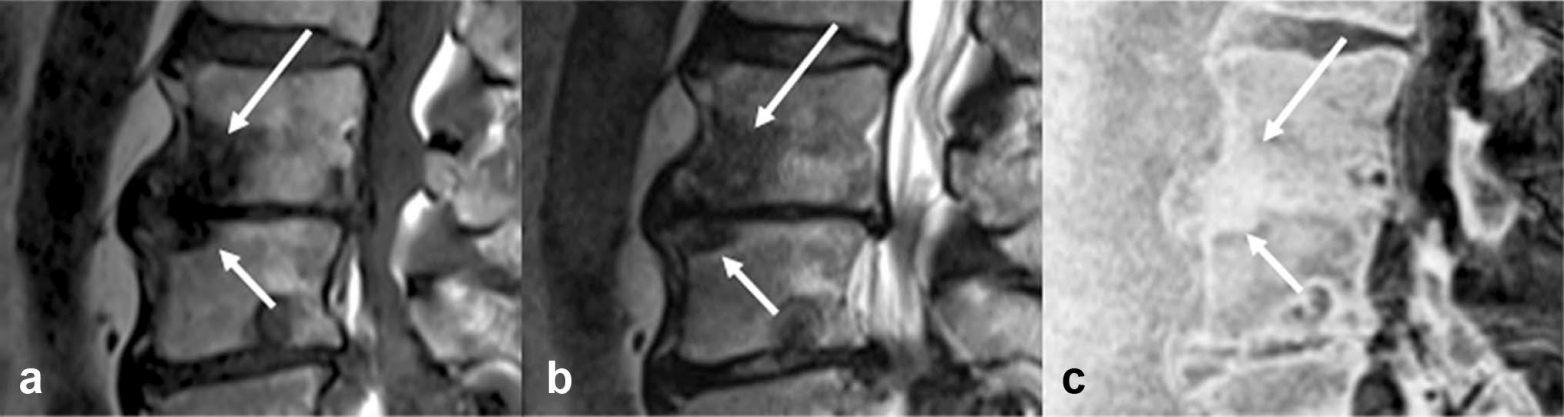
Modic type 3 change. Modic type 2 change represents subchondral bony sclerosis. Sagittal T1-weighted (a) and T2-weighted (b) MR images of an 84-year-old male patient show areas of hypointensity (arrows). Sagittal MR bone image c shows sclerotic changes as hyperintense areas (arrows)
